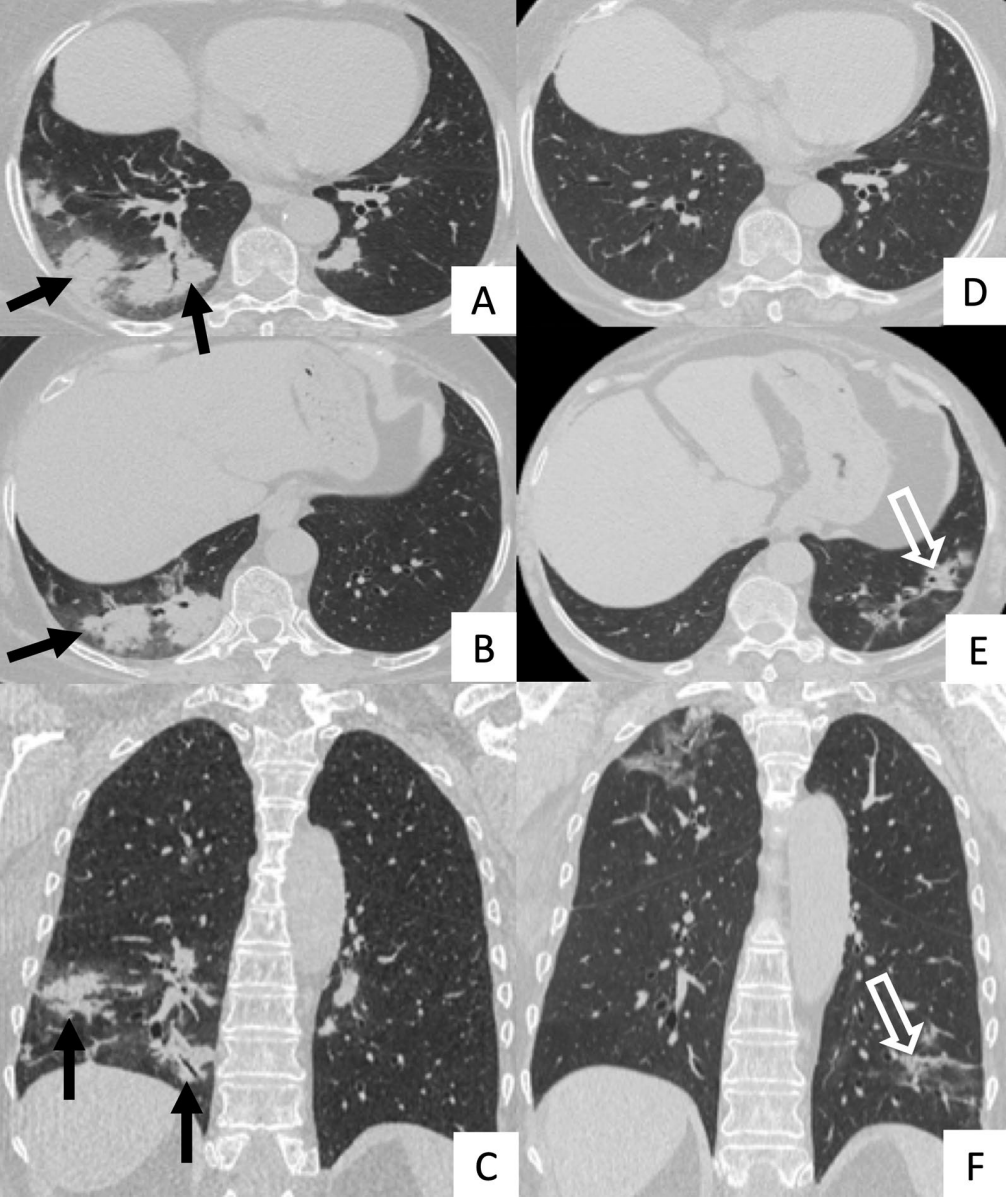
Axial (A, B) and coronal (C) HRCT of a PM-DM patient, showing OP pattern. A–C Peripheral and bronchocentric consolidations (black arrows); the “air bronchogram sign” is clearly recognizable. Follow-up HRCT images (D–F) demonstrate the typical evolution of organizing form, with tendency to migration and location changing (empty white arrows)
